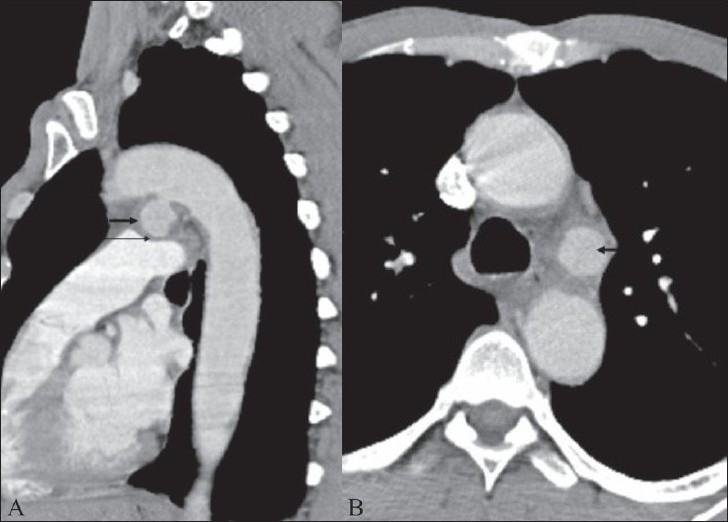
Sagittal reformatted (A) and axial (B) contrastenhanced CT scan of the chest shows a wide-necked luminal outpouching along the inferior aspect of the aortic arch (thick arrow) in the aorto-pulmonary window. The main pulmonary artery shows mild dilatation and indentation by the aneurysm in its distal part (thin arrow). The thoracic aorta shows normal caliber without any significant atherosclerotic changes. The ‘three-vessel’ appearance seen in B is also referred to as the ‘triple-star sign.’
